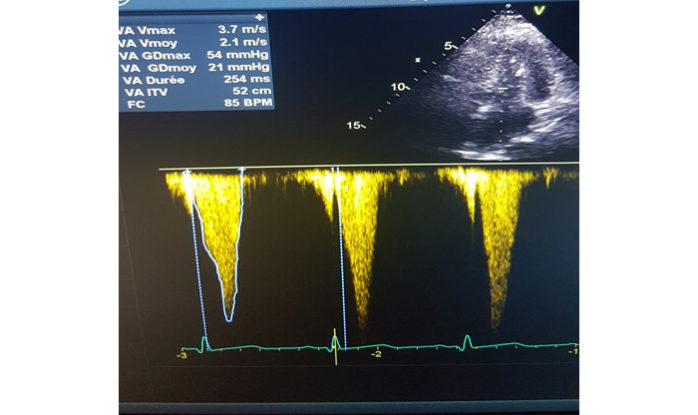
Apical 5 cavities view of transthoracic echocardiography. Diagnostic of left ventricular outflow tract obstruction is assessed here by a maximal pressure gradient measured at 54 mmHg. Velocity peak is maximum in telesystolic.
